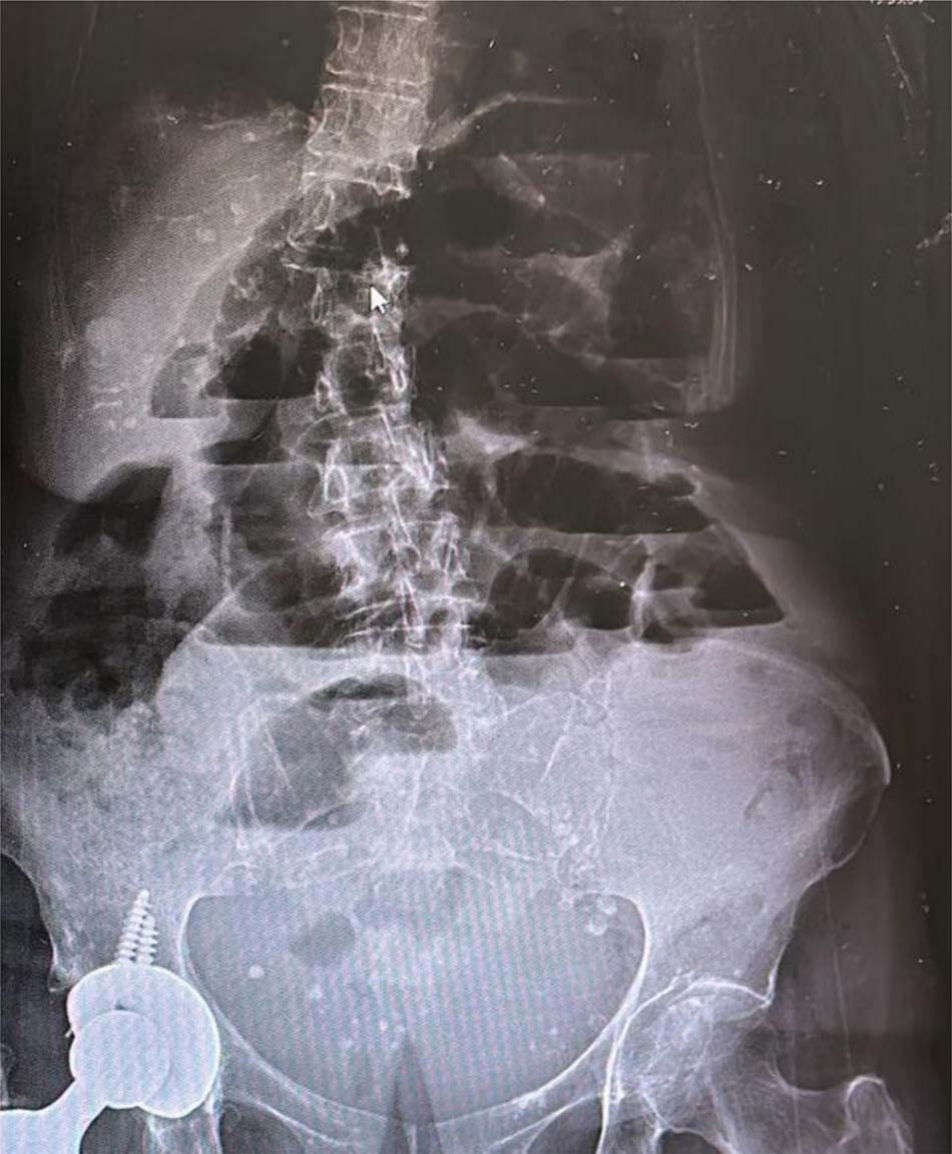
Abdominal radiography with air-fluid levels. Dilation of intestinal loops with multiple air-fluid levels.
